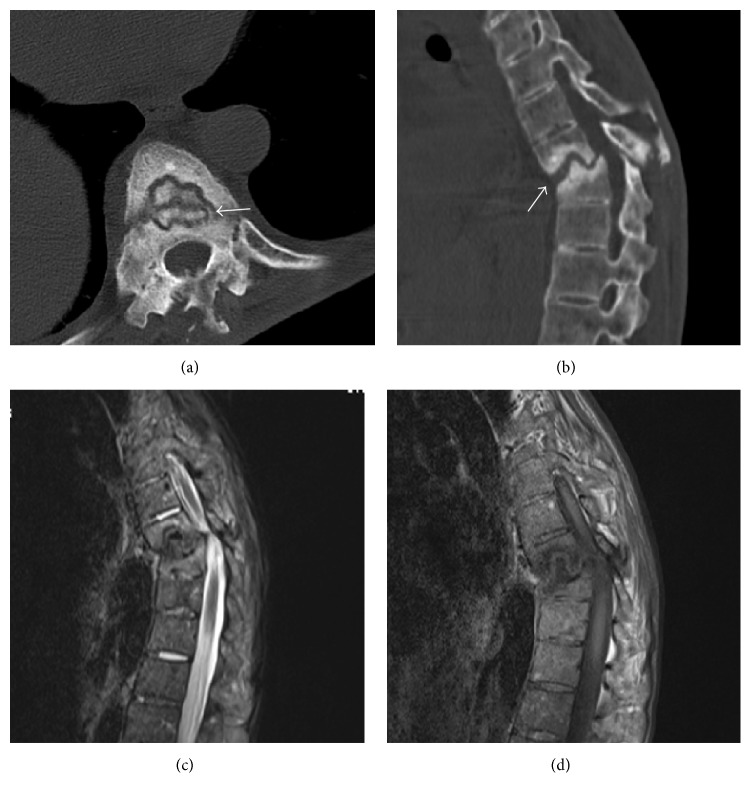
A 54-year-old female patient presented with painful round kyphosis and motor loss of bilateral lower extremities. CT scan showed destruction of the vertebral body and vacuum phenomenon (a, arrow). CT sagittal reconstruction image (b) demonstrated irregular bony gap (arrow) from anterior to posterior column and sclerosis of adjacent vertebral bodies. T2- (c) and T1-weighted (d) sagittal images revealed low signal at the pseudarthrosis and compression of the spinal cord.
